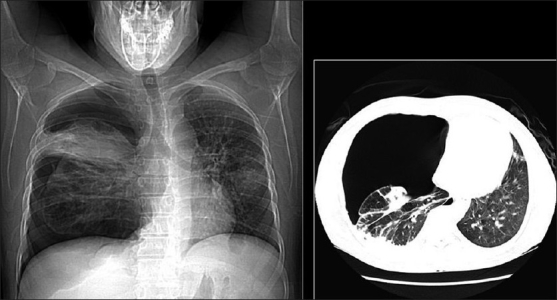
Pneumocystis carinii* pneumonia. Chest X-ray and computed tomography show a left-sided ground-glass pattern and a right-sided large tension pneumothorax. Note the mediastinal shift
